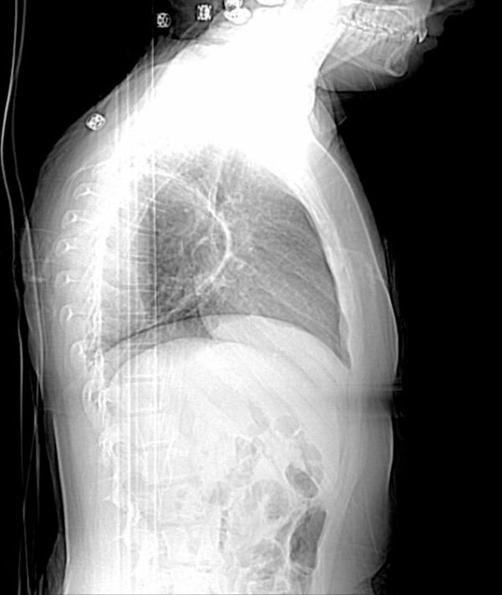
Initial lateral view chest X-ray.
